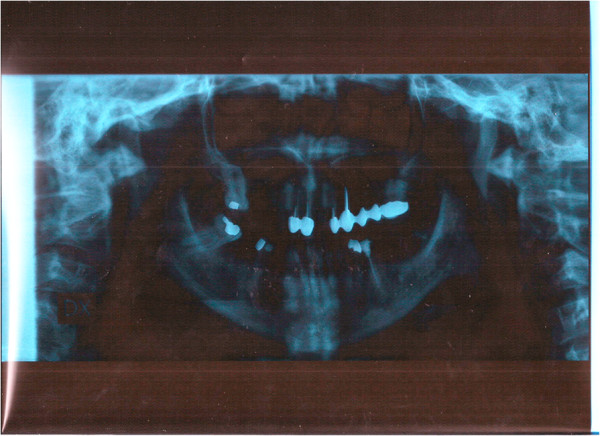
Preoperative orthopanoramic X-ray.
